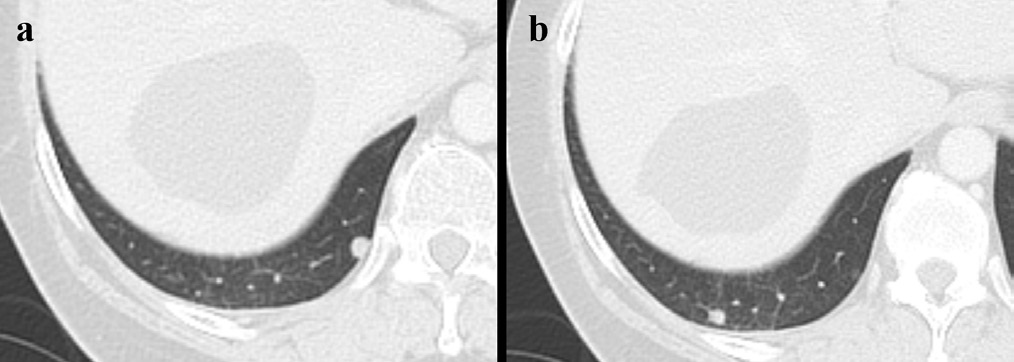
Chest computed tomography performed 5 weeks postoperatively. (a, b) Axial image shows two nodules in the lower lobe of the right lung (each nodule size is 5 mm)
